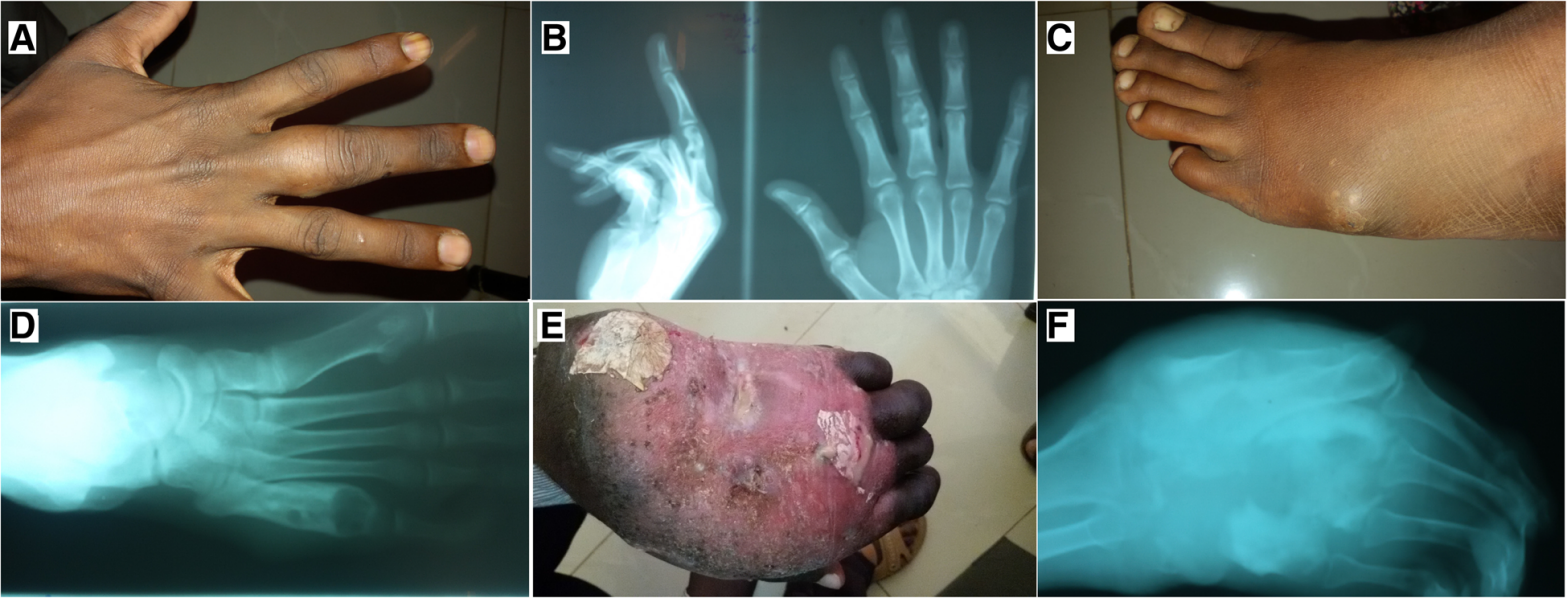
Pre-amputation images of the finger, toe, and hand with X-ray showing mycetoma. a Right middle finger eumycetoma (proximal phalanx). b PA and lateral X-ray view of the same patients showing cavity and evidence of mycetoma (this patient treated by finger Ray’s amputation). c Left toe mycetoma with sinus cavity. d X-ray was showing cavity and mycetoma (this patient treated by toe Ray’s amputation). e Hand showing mycetoma deformity, discoloration, and loss of function. f X-ray of the same patient was showing a deformity, cavitation, and mycetoma (this patient treated by below-elbow amputation)
